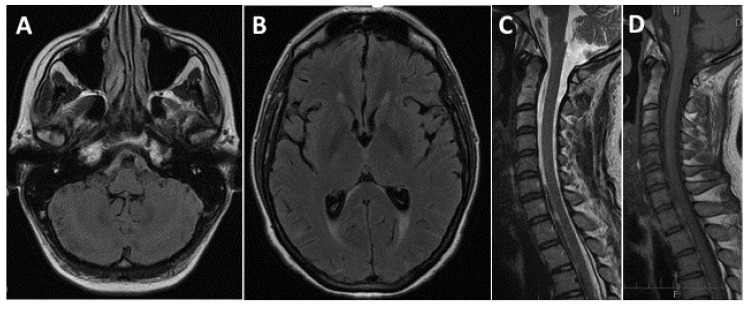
Initial MRI, subarachnoid hemorrhage. (A) Axial FLAIR image, premedullary subarachnoid hemorrhage (SAH). (B) Axial FLAIR image, SAH on the posterior convexity. (C) Sagittal T2—weighted image, premedullary SAH. (D) Sagittal T1—weighted image, premedullary SAH.
